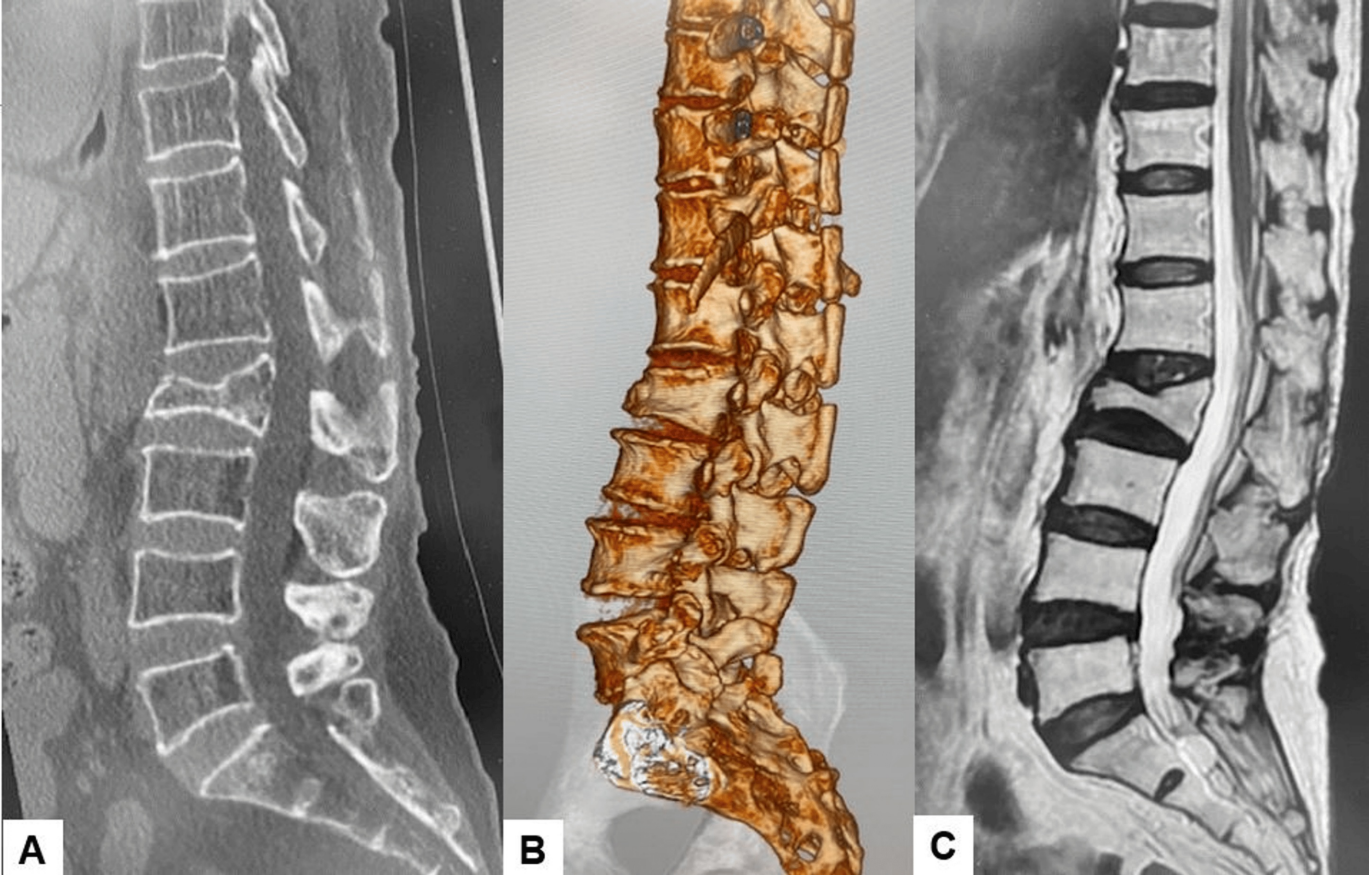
Preoperative CT and MRI (A) Mid-sagittal reconstruction CT. (B) Three-dimensional reconstruction CT. (C) T2-weighted mid-sagittal MRI.
